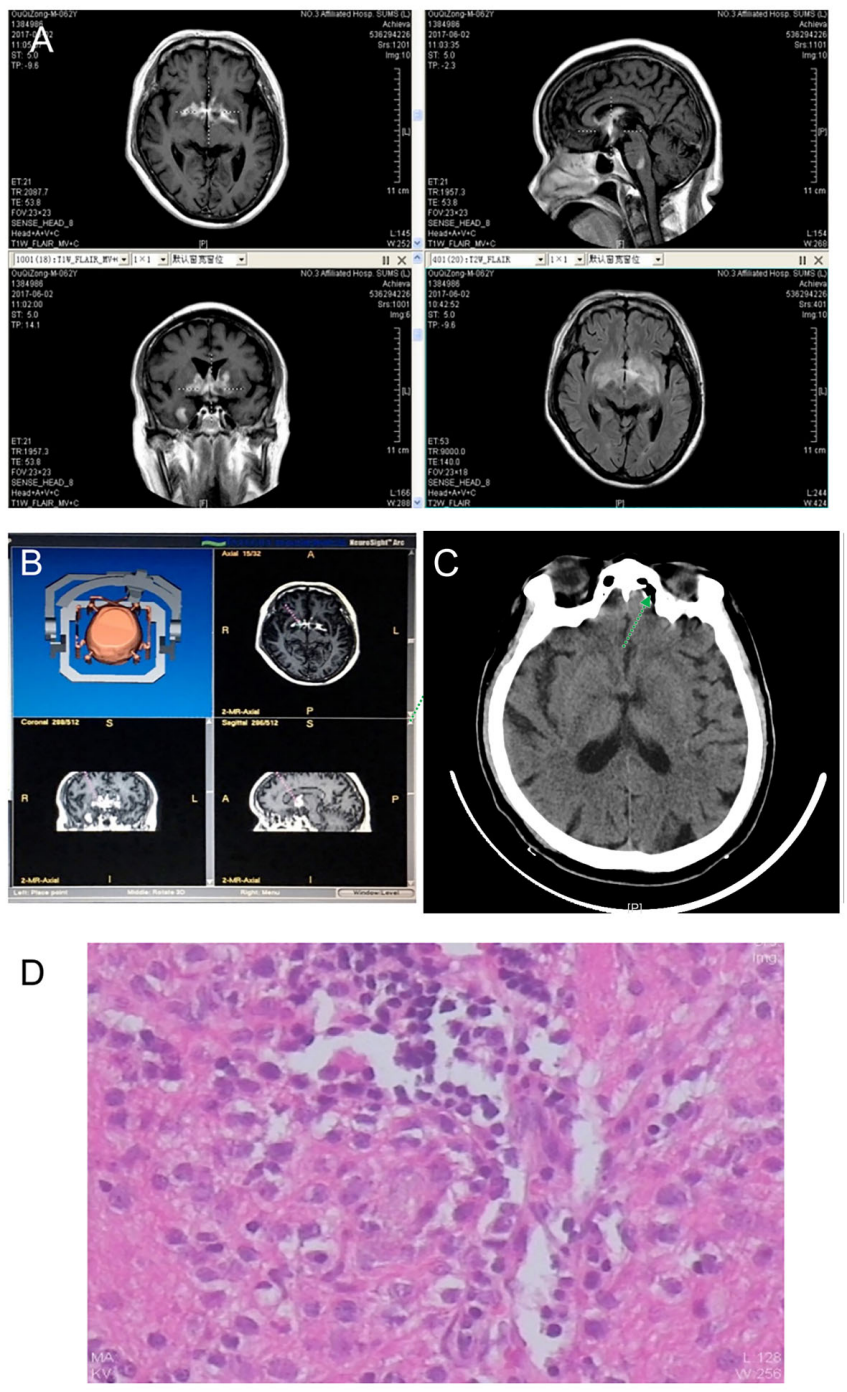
Case 2. A, T1-weighted contrast-enhanced magnetic resonance imaging (MRI) showed a bilateral internal capsule, corpus callosum, and a high-intensity lesion in pons-medulla junction. MRI T2 flair showed an abnormally high signal. B, Three-dimensional surgical plan - right internal capsule target. C, Computed tomography 1 day after surgery; the arrow shows small areas of pneumocephalus in the genu of the right internal capsule. D, Pathological diagnosis of diffuse large B-cell lymphoma.
